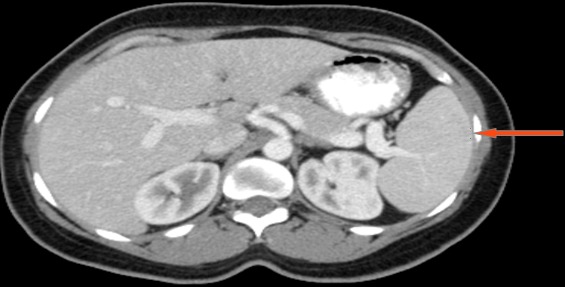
Pre-surgery axial abdomen CT: shows spleen (red arrow)
